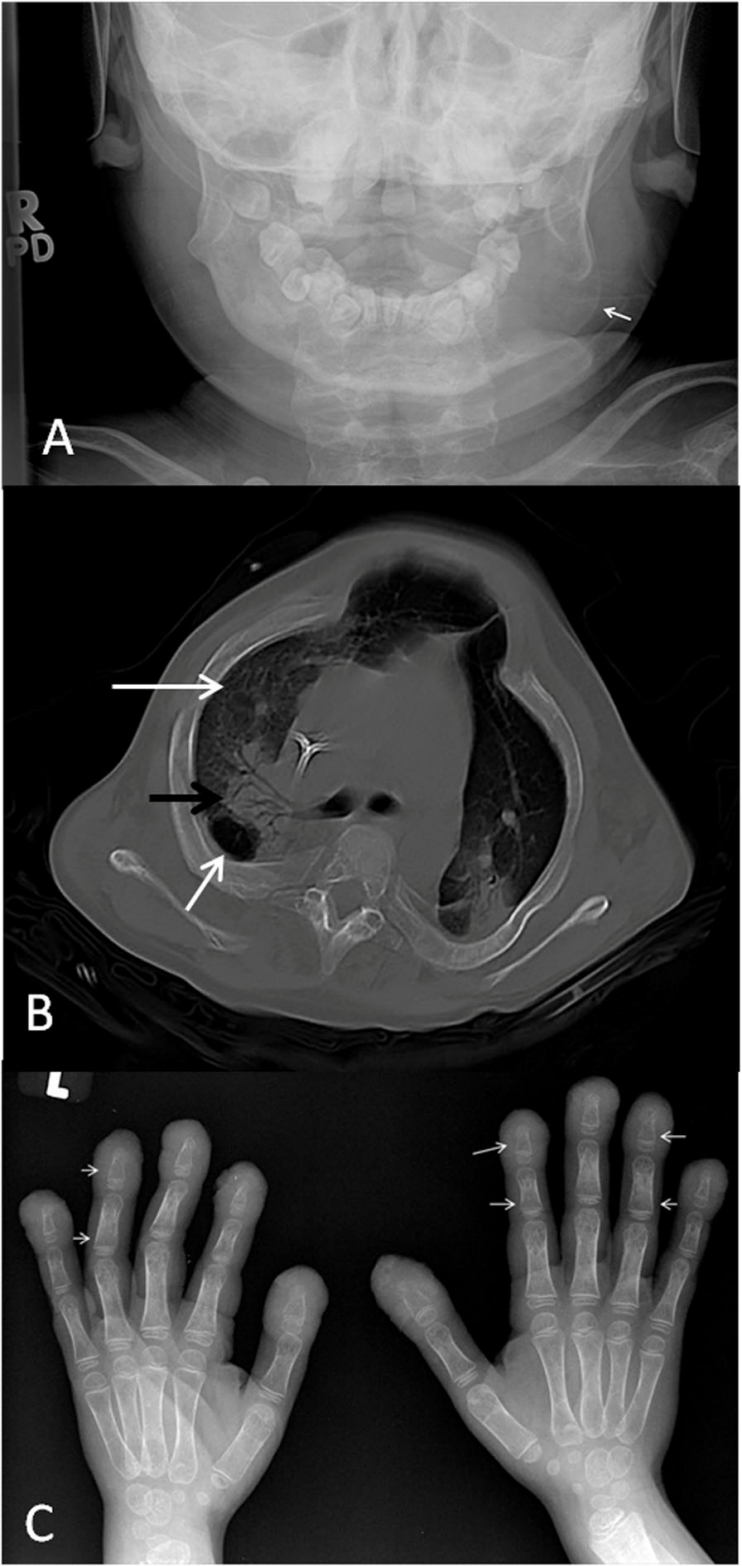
Several imaging findings in a male patient with deficiency of interleukin 1 receptor antagonist (DIRA). a Posteroanterior view of the mandible at the age of 7 years when he presented with left mandibular swelling shows a well-defined lytic lesion within the body of the left mandible with expansion of the bone and thinning of the cortex (arrow). There are no aggressive features. b Axial unenhanced CT of the chest in bone window demonstrates diffuse broadening of the ribs with ground glass density of the medulla and thinning of the cortex. Bone biopsy showed fibrous changes indicative of healing that is likely related to the patient’s bone disease. There are alveolar (black arrow) and interstitial (long white arrow) pulmonary opacities and nodules with cyst formation (short white arrow). Lung biopsy: pulmonary hemosiderosis. c Anteroposterior radiograph of the hands performed when the patient presented with abnormal nails demonstrates multiple bilateral symmetrical metaphyseal lytic lesions with associated cortical thinning (arrows). There is distal phalangeal soft tissue thickening
